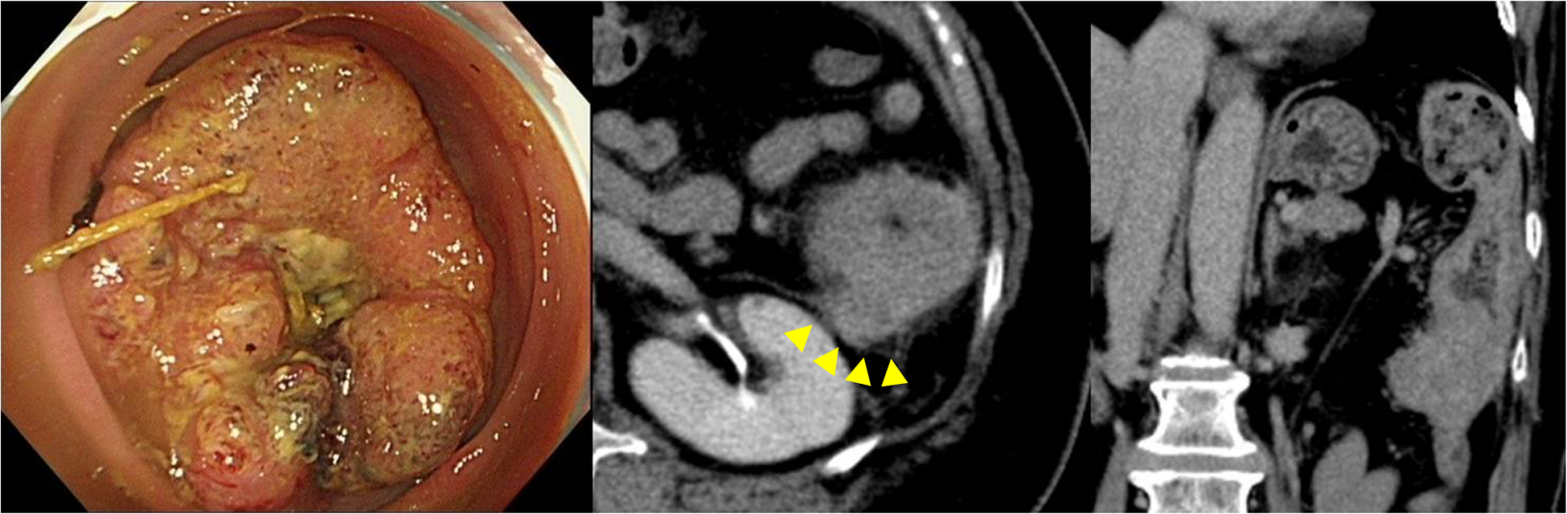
Colonoscopy and computed tomography: circumferential T4 descending colon cancer invading the Gerota’s fascia (arrow head) at the anal side of the splenic flexure
